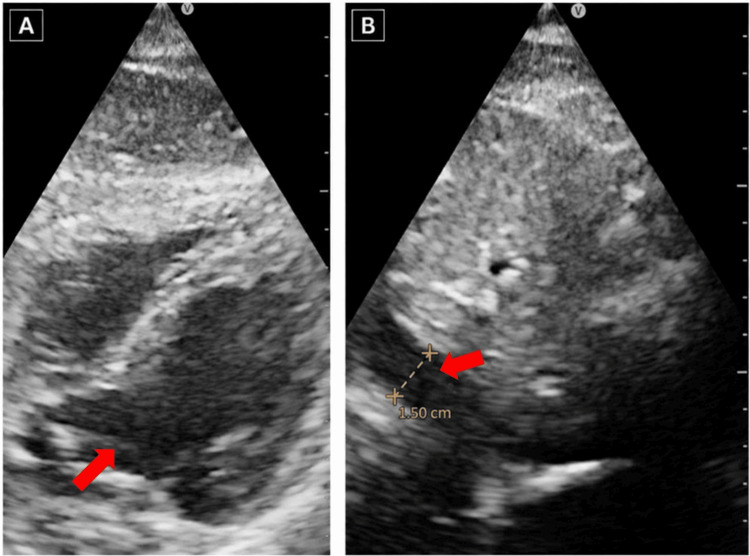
Transthoracic echocardiography demonstrating left ventricular systolic dysfunction and normal inferior vena cava dynamics (A) Echocardiographic view showing global left ventricular hypokinesia; the red arrow indicates the left ventricular cavity and myocardium assessed for global systolic dysfunction. (B) Subcostal inferior vena cava view showing a normal inferior vena cava diameter, measured at approximately 1.50 cm, with preserved respiratory variation; the red arrow indicates the measured inferior vena cava segment
